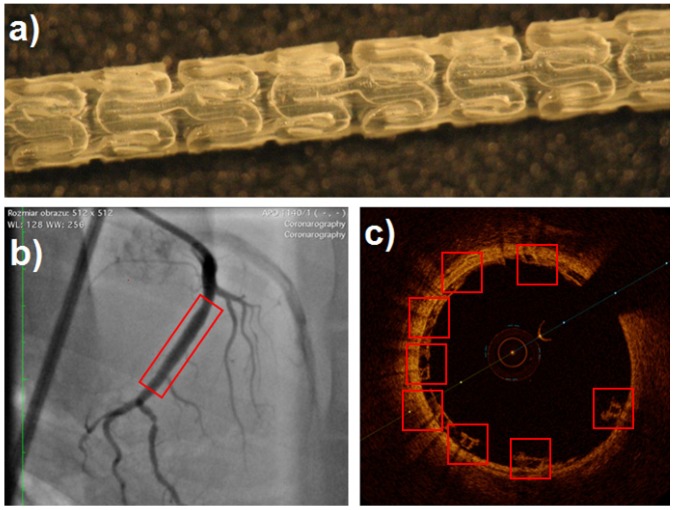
One of the test variants of a bioresorbable coronary stent developed with the use of the proposed constitutive methodology within the Apollo STRATEGMED2 project: (a) Stent after cutting and free compression; (b) area of clinical test implementation (angiography image); (c) area of clinical test implementation (intravascular ultrasound image).
